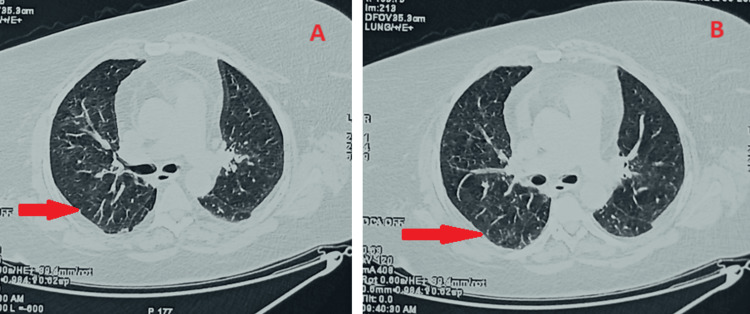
Chest CT images obtained after the patient underwent corticosteroid therapy revealed minor reticulations (indicated by red arrows) along with a noticeable reduction in frosted glass appearance.
